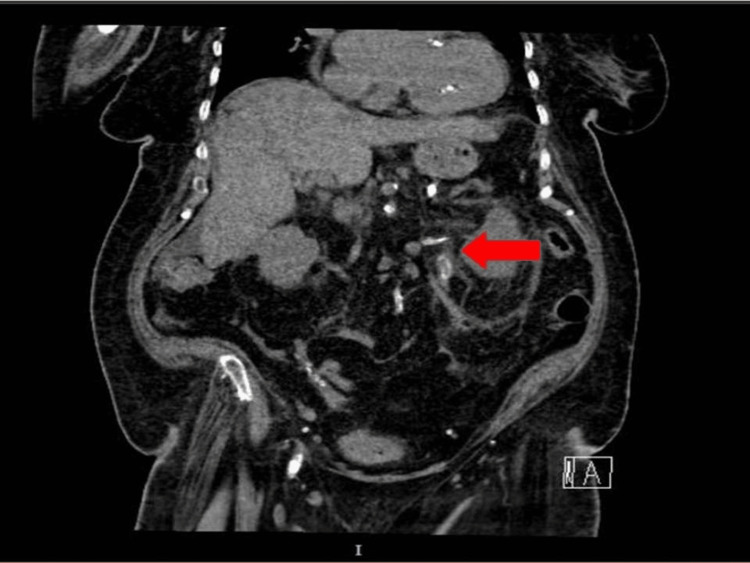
Non-contrast CT showing a calcified renal papilla in the left proximal ureter (arrow).
